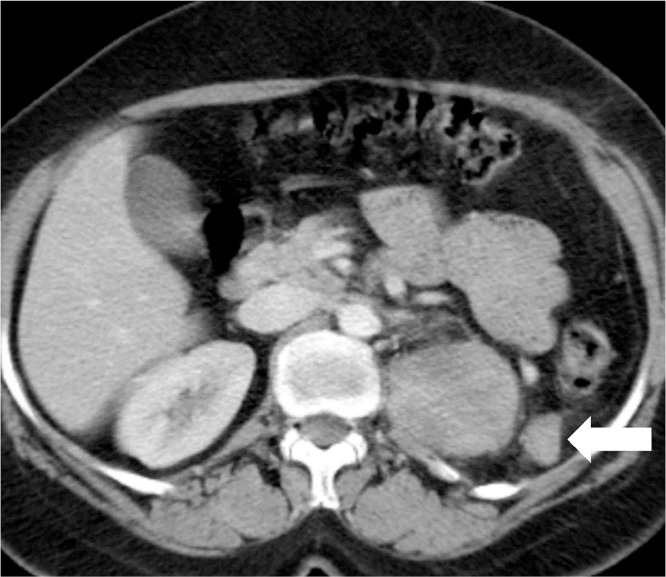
Three-month follow-up CT scan showing interval development of enhancing peritoneal deposit (white arrow) near soft tissue mass in the left nephrectomy bed.
